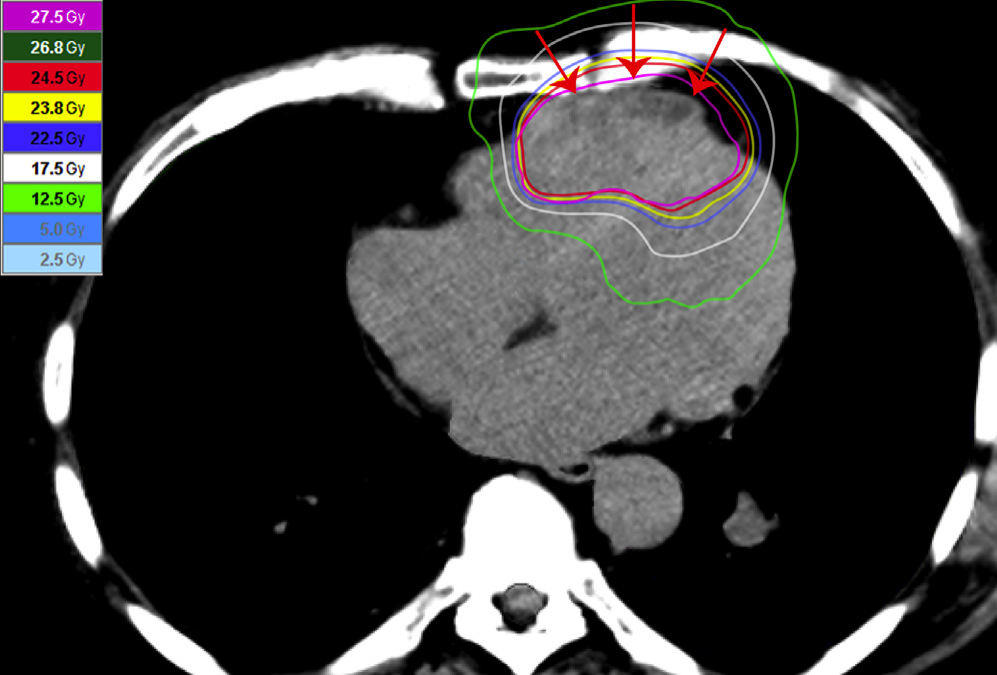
Computed Tomography and Radiotherapy Plan The masses (arrows) infiltrate the free wall and the apex of the right ventricle. The colored lines contour the intensity-modulated radiation dose (see scale on the left). The planned volume takes into account the cardiac motion to keep the metastases within the radiation field throughout the cardiac cycle.
